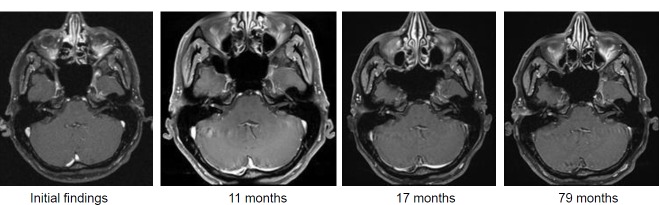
Axial MRI, T1–w + CM; constant size of a vestibular schwannoma during a follow-up of 6.5 years.
